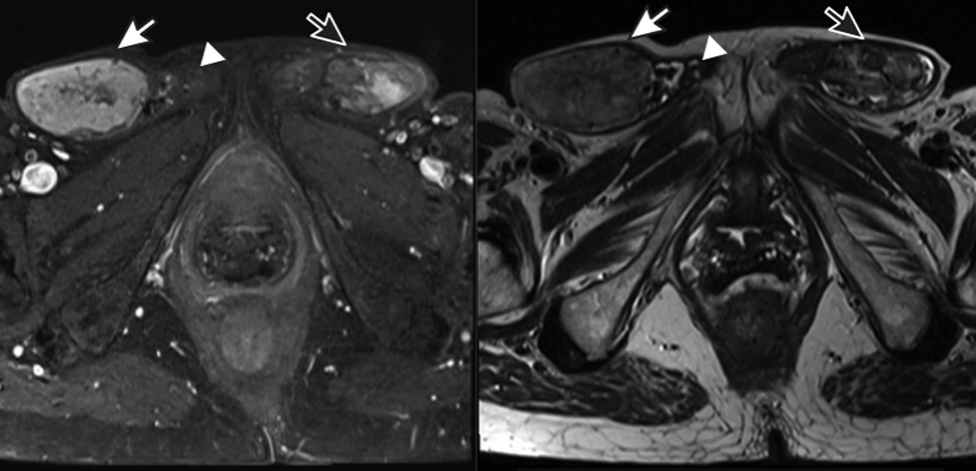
T1- and T2-weighted magnetic resonance imaging (MRI) transverse section of pelvis showing bilateral testis. White arrow showing the right testis, white arrow head showing the right epididymis, and the black arrow with white outline showing the left testis.
